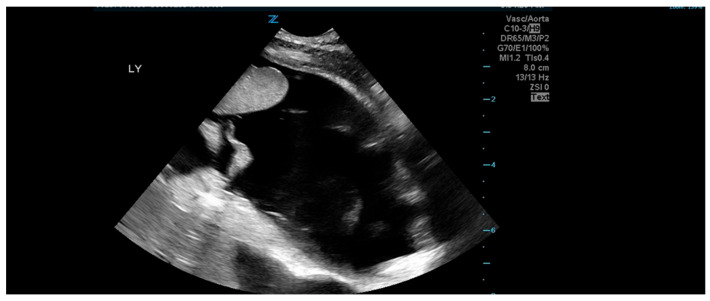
Sonographic imaging of the ascites.
